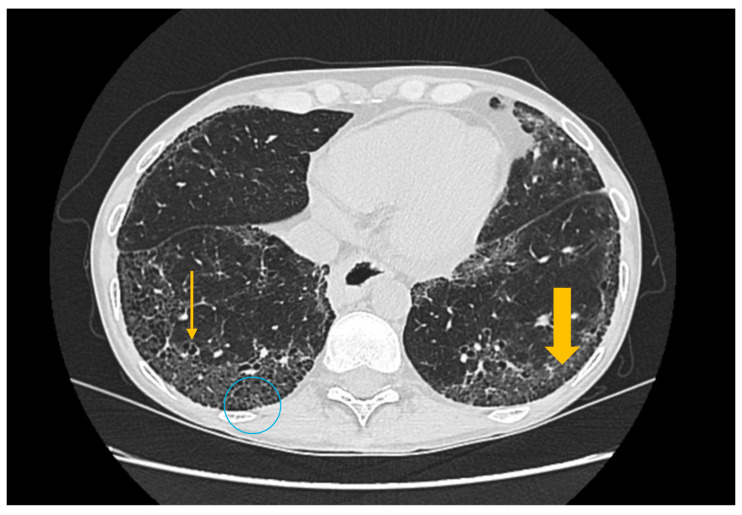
High-resolution computed tomography (HRCT) of the chest in usual interstitial pneumonia (UIP). Axial HRCT image demonstrates subpleural reticulations (thick arrow), traction bronchiectasis (thin arrow), and honeycombing (circle), with an asymmetric distribution and basal predominance, findings characteristic of UIP.
